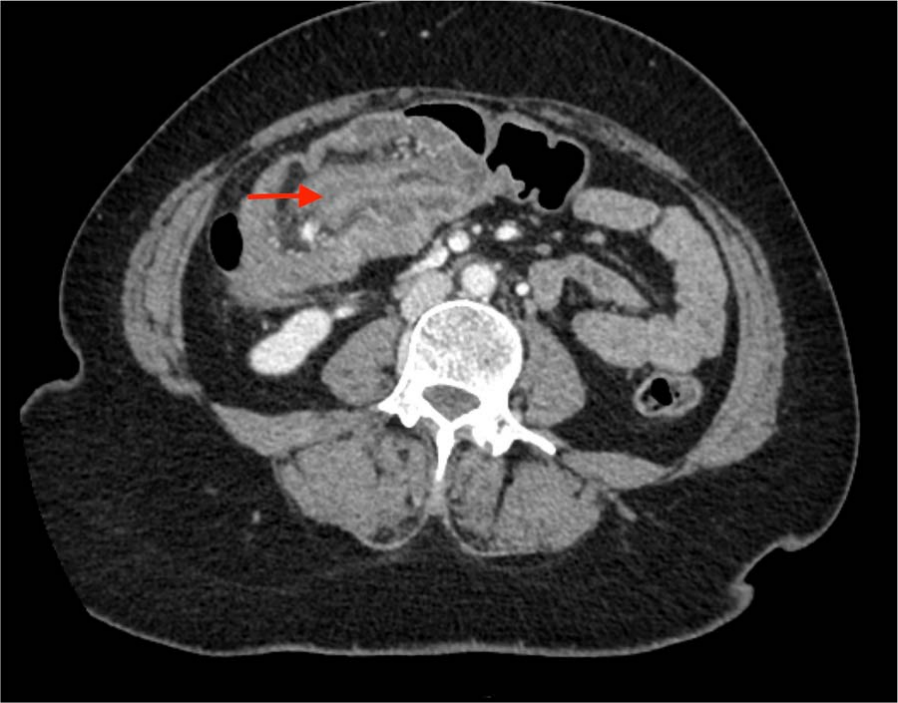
Contrast enhanced CT of the abdomen showing bull’s eye sign (arrow) suggestive of ileocolic intussusception.
